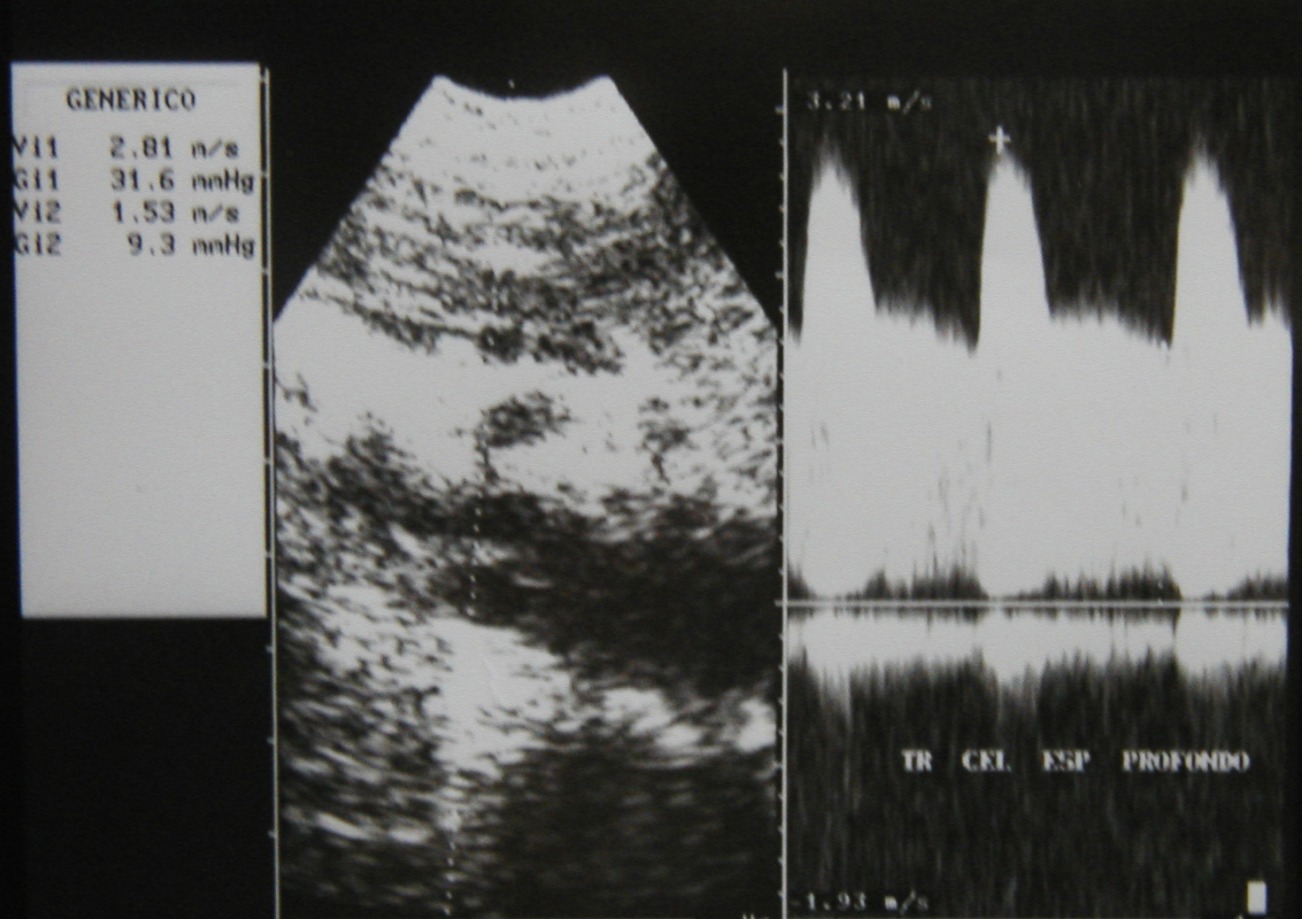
Continuous Doppler of Celiac Artery Stenosis, deep expiration.
